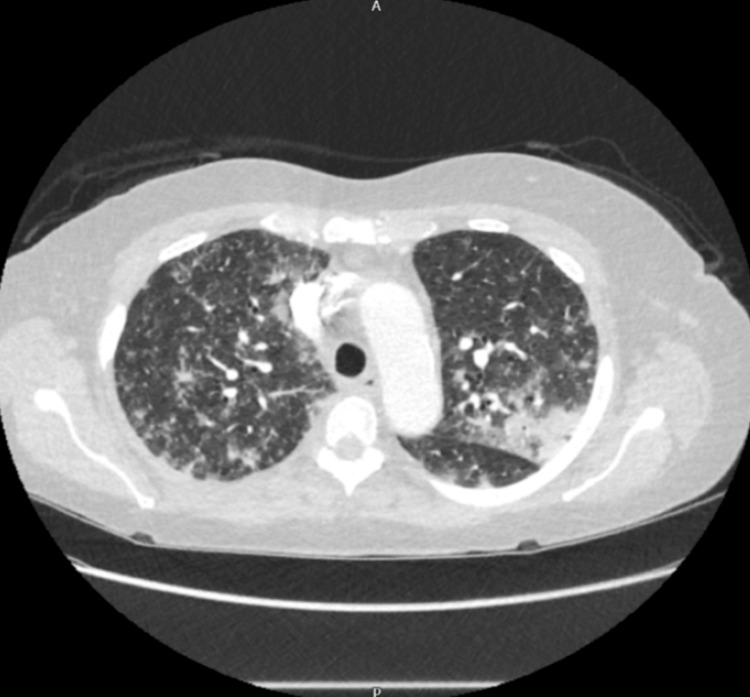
CTPA showing patchy bilateral consolidation and inflammatory changes at the mid zone level. CTPA: CT pulmonary angiography
